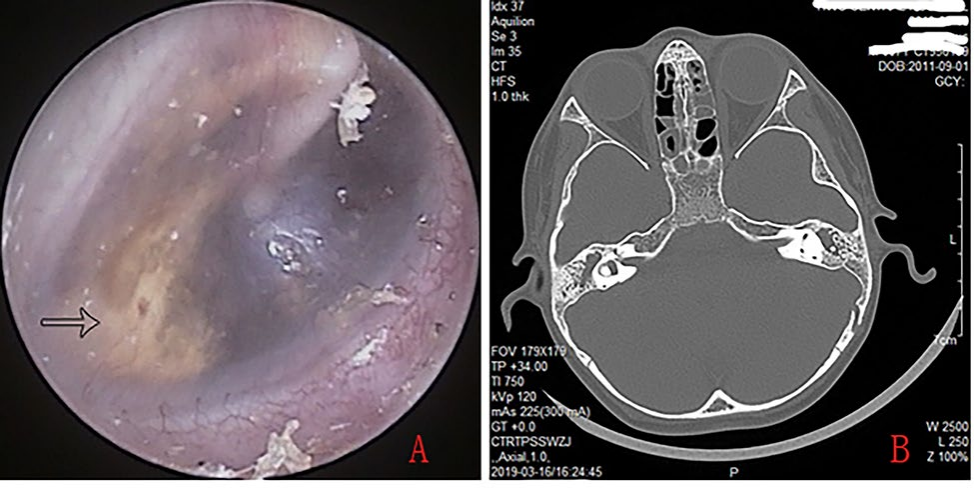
A-8 year-old boy. Endoscopic examination showed an right intact blue eardrums and yellow granulation tissue of tympanic cavity (white arrows) (A), CT revealed the bilateral soft tissue density in the antrum, aditus ad antrum and mastoid (B)
